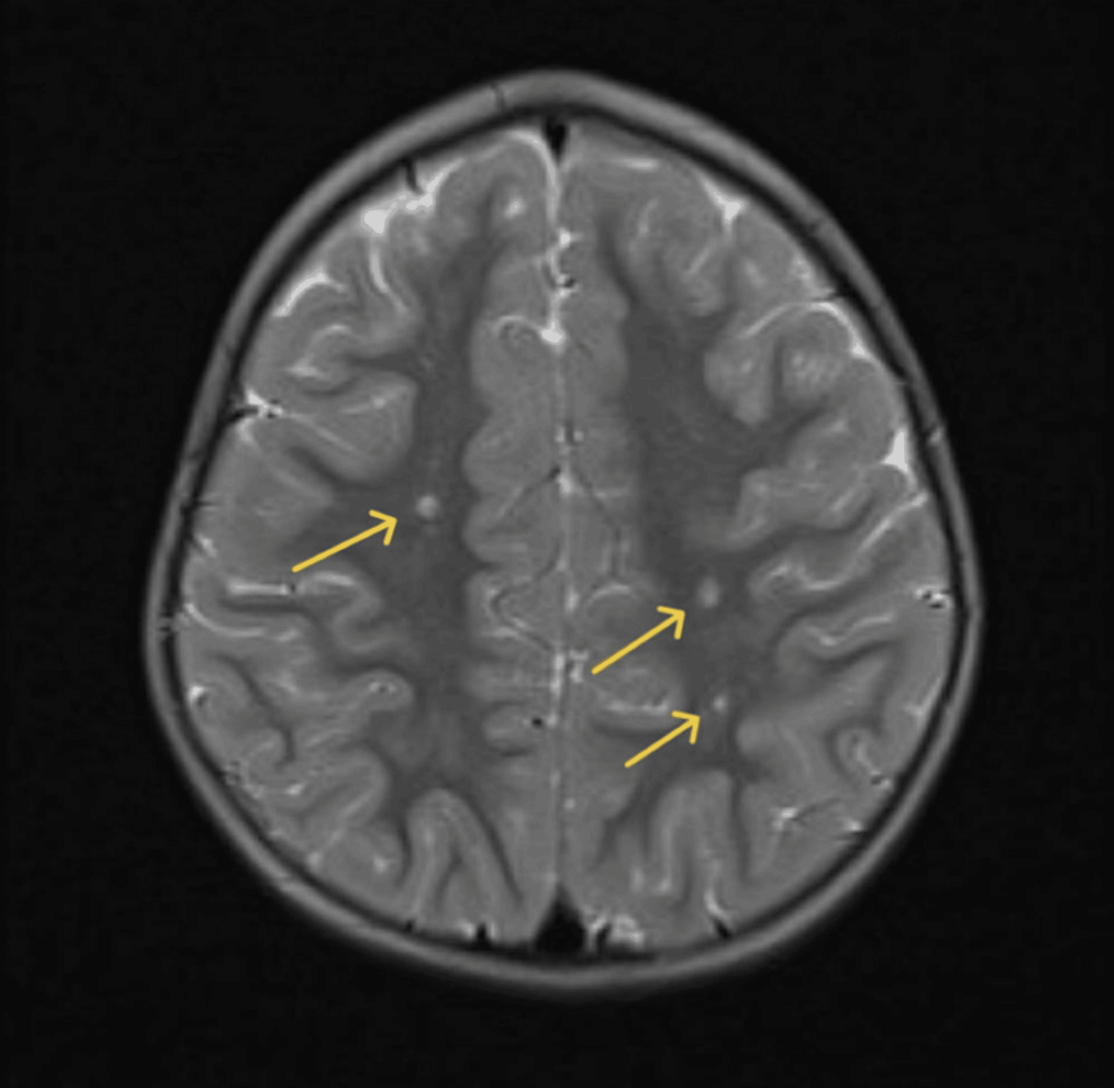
Initial MRI scan: dilated perivascular spaces Axial T2-weighted MRI demonstrating multiple dilated perivascular (Virchow-Robin) spaces within the parasagittal deep and subcortical white matter of bilateral parietal regions. Lesions are well-circumscribed, with CSF-like signal intensity, without surrounding gliosis, mass effect, or cortical involvement (arrows).
